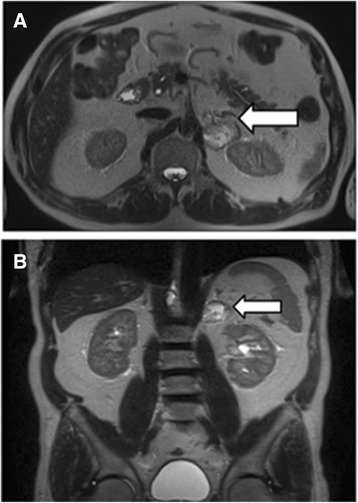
Abdominal MRI scan. a Coronal MRI scan of the abdomen. b Axial MRI scan of the abdomen. The *arrows* indicate the surrenal mass: the signal was highly inhomogeneous and the central area was partially fluid
